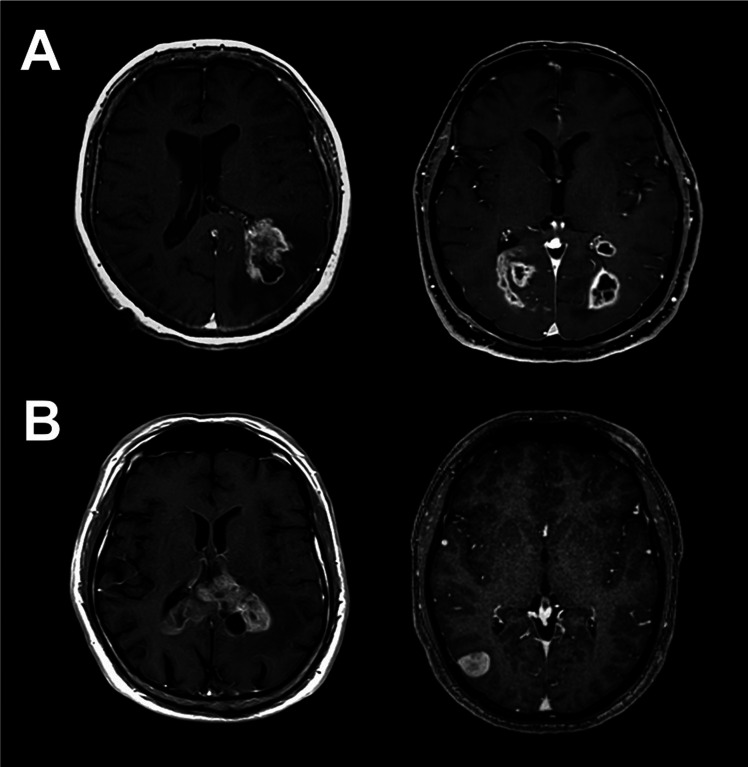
Representative MRI scans of patients with GB. (A) GB with unifocal (left) or multifocal (right) growth pattern. (B) GB with (left) or without (right) SVZ involvement. MRI, magnetic resonance imaging; GB, glioblastoma; SVZ, subventricular zone.
